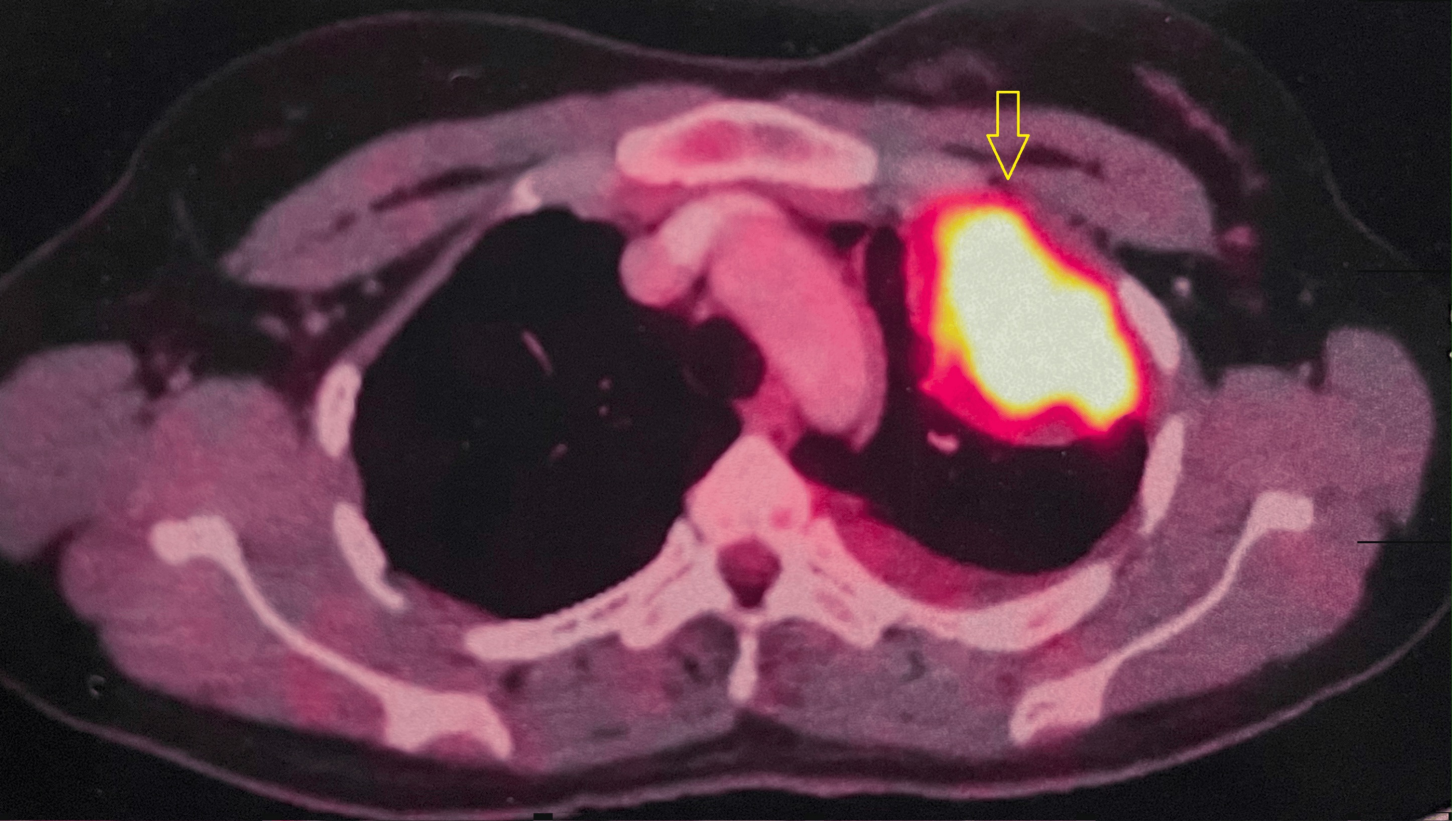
PET-CT scan showing FDG uptake in the left upper lobe PET-CT = positron emission tomography-computed tomography scan; FDG = fluorodeoxyglucose
